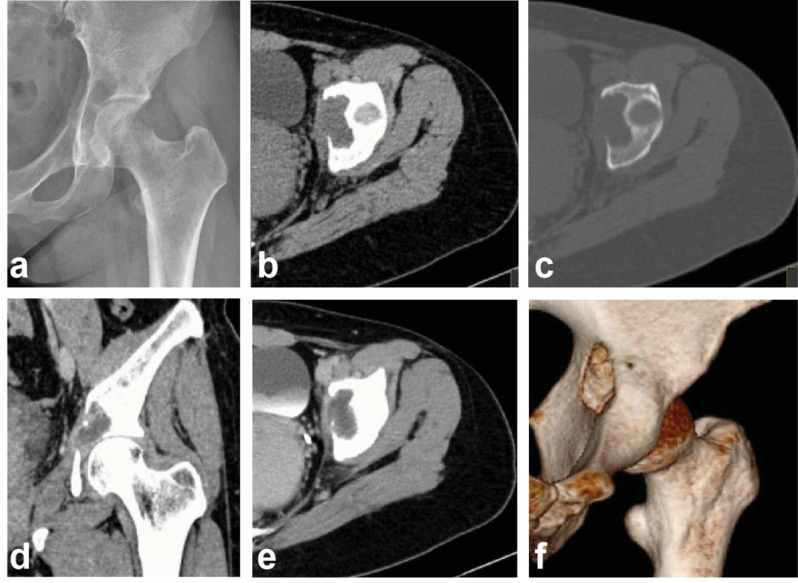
(a) Left hip joint radiograph. Lytic lesion was visualized in the left acetabulum, and the continuity of the local cortical bone was interrupted. Left hip joint CT: (b) Axial soft tissue window. (c) Axial bone window. (d) Coronal contrast-enhanced CT. (e) Axial contrast-enhanced CT. (f) Three-dimensional reconstruction. Osteolytic bone destruction and a soft tissue mass were observed in the left acetabulum. The wall was strengthened and there was a no enhancement liquid low-density shadow in the mass.
